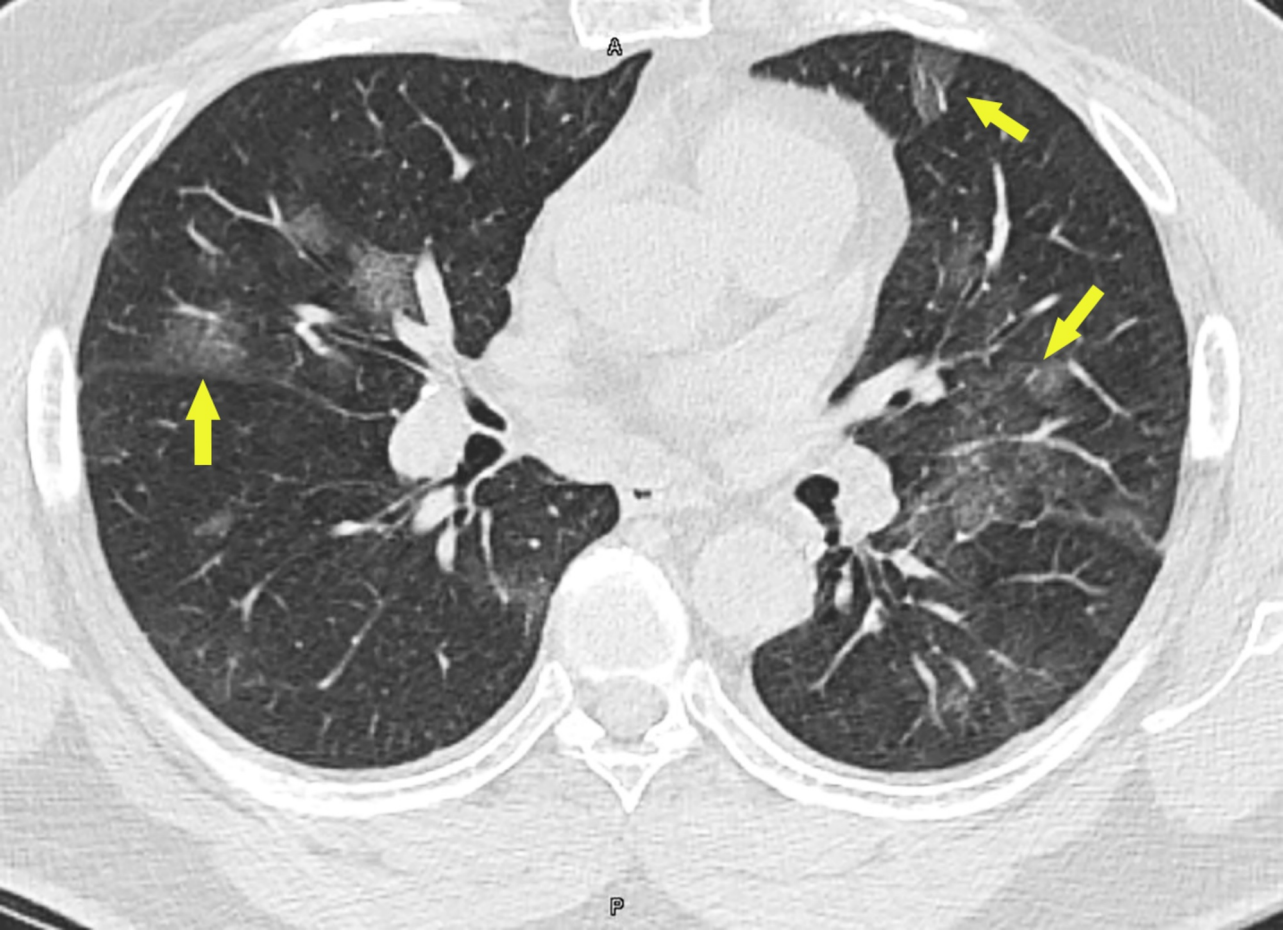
CT scan of the thorax showing multifocal bilateral ground-glass lung opacities with areas of subpleural sparing (arrows)
